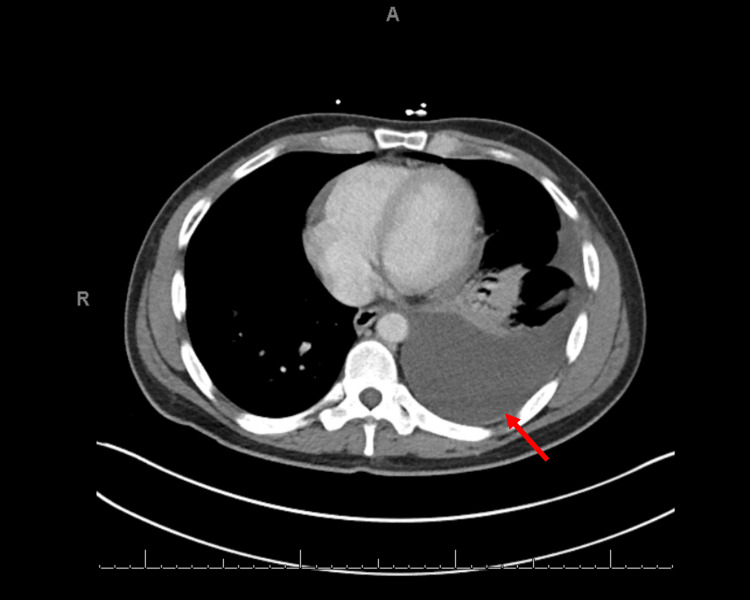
Computed tomography of the chest demonstrating a large left pleural effusion (red arrow) noted on admission.
